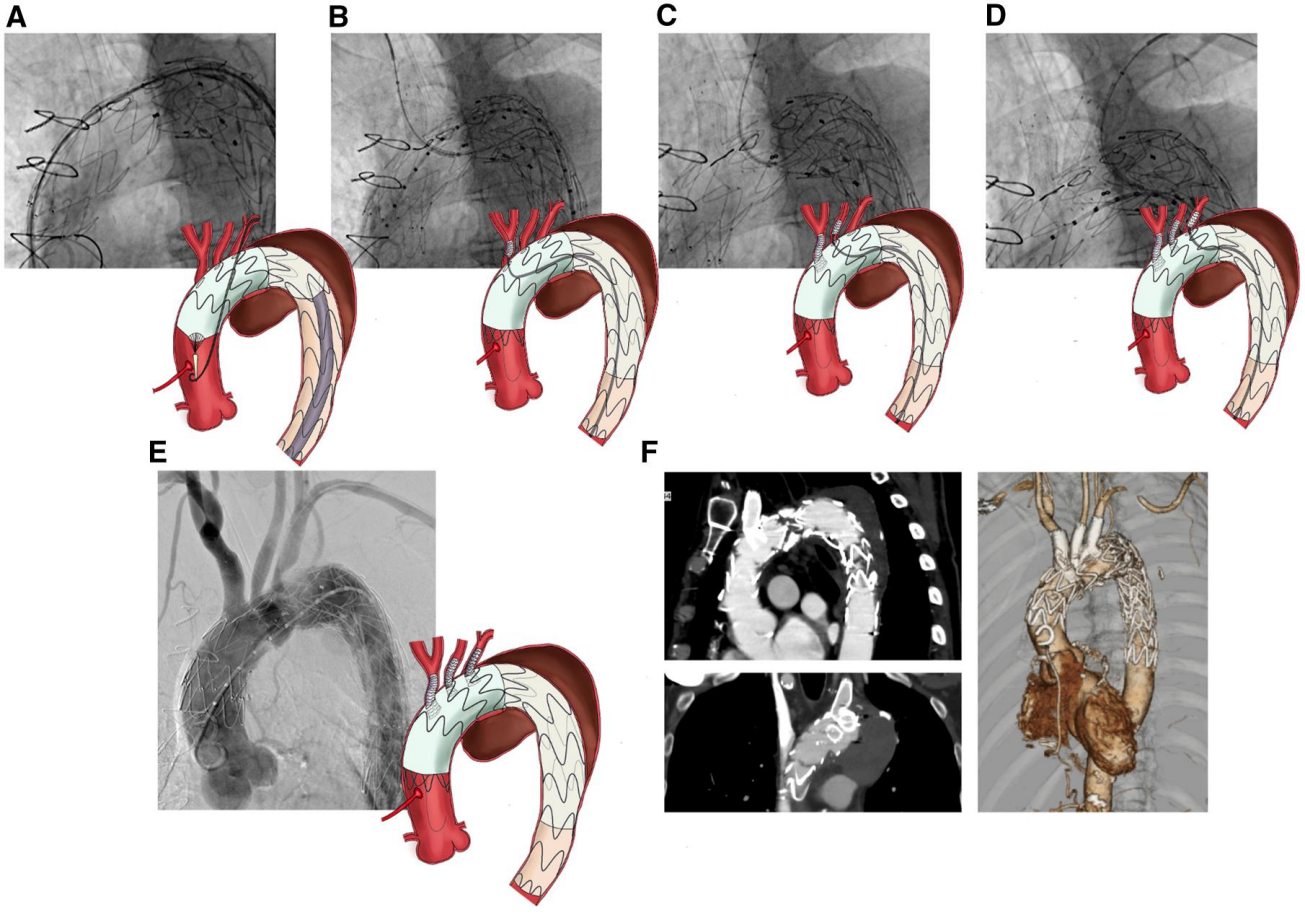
Stepwise implantation using transfemoral bridging stent-graft delivery with postoperative imaging. (A) Fenestrated physician-modified endograft (f-PMEG) was deployed with the radiopaque marker positioned slightly more centrally than the cervical branch. (B) A pull-through wire was inserted between the right brachial artery and left femoral artery, and a bridging covered stent (VIABAHN, 13 mm × 5 cm) was deployed. (C) A pull-through wire was inserted between the left common carotid artery and left femoral artery, and a bridging covered stent (VIABAHN, 10 mm × 5 cm) was deployed. (D) A pull-through wire was inserted between the left brachial artery and left femoral artery, and a bridging covered stent (VIABAHN, 13 mm × 5 cm) was deployed. (E) Aortography showing leakage of contrast medium into the aneurysm (a type 4 endoleak). (F) Follow-up computed tomography (CT) on postoperative day 6 showing no endoleaks or abnormalities of the supra-aortic branches
